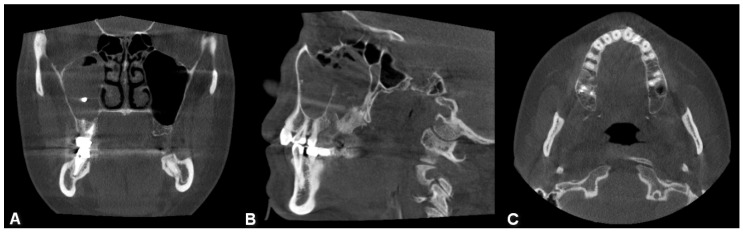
CBCT image of unilateral maxillary sinusitis and oro-antral fistula with the characteristic radiological image of fungal infection, which radiologically mimics foreign bodies ((A): coronal, (B): axial, (C): sagittal view).
